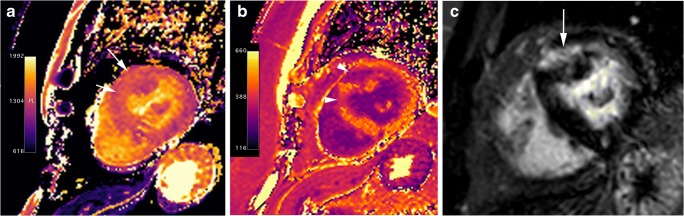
T1 mapping in a 67-year-old woman with basal anterior and anteroseptal HCM. a, Short-axis nonenhanced MOLLI (1.5 T) T1 map shows prolonged T1 values in the area of myocardial hypertrophy (arrows). b, T1 map obtained at LGE MR imaging shows abnormal shortening of the T1 values in the hypertrophied segments (arrowheads). The extracellular volume (ECV) for the hypertrophied segments was abnormally elevated (44,6 vs 29% for the lateral wall) (haematocrit, 0.42). Normal ECV values of 25.3 ± 3.5% (1.5 T). c, LGE MR image through the same plane shows midwall enhancement in the hypertrophied segments (arrow)
